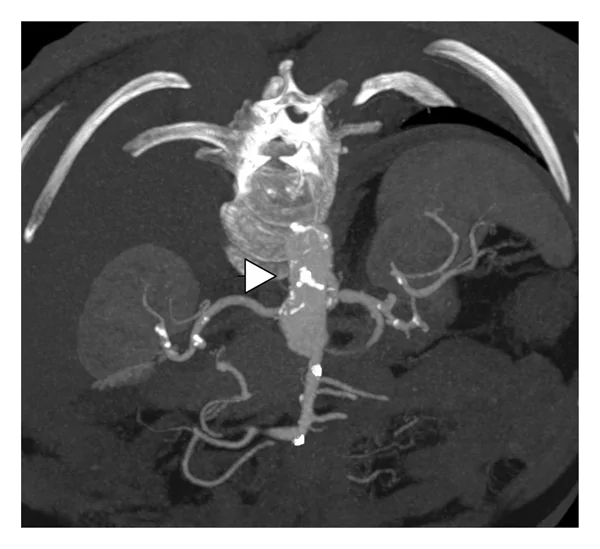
A contrast‐enhanced axial abdomen CT angiography MIP scan exhibiting calcific plaques not only in the abdominal aorta and SMA but also in the bilateral renal arteries. Widespread calcifications suggest extensive atherosclerosis affecting multiple major abdominal vessels, which can lead to reduced blood flow to vital organs, such as the kidneys and intestines.
